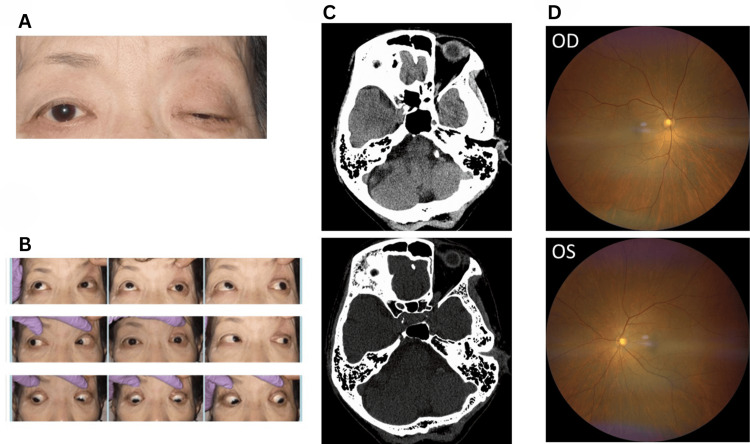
Ophthalmic examination findings and CT scanning images at initial visit (A) Photograph of the eyes and (B) eye movement assessment at the initial evaluation. The patient exhibited left eye ptosis and restricted upward movement of the left eye. (C) CT scan images showing no aneurysm in the internal carotid artery-posterior communicating artery region. (D) Fundoscopic examination of both eyes revealed no significant abnormalities. CT: computed tomography, OD: oculus dexter (right eye), OS: oculus sinister (left eye)
